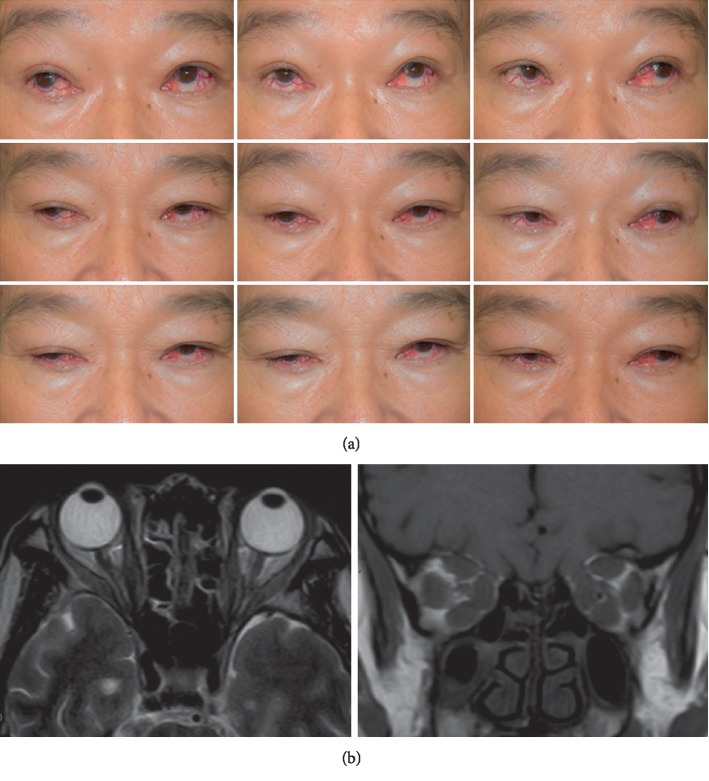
One case diagnosed with bilateral dysthyroid optic neuropathy (DON). (a) Eye movement testing showed limited eye movement and strabismus in both eyes. (b) Magnetic resonance imaging (MRI) showed enlargement of extraocular muscles.
